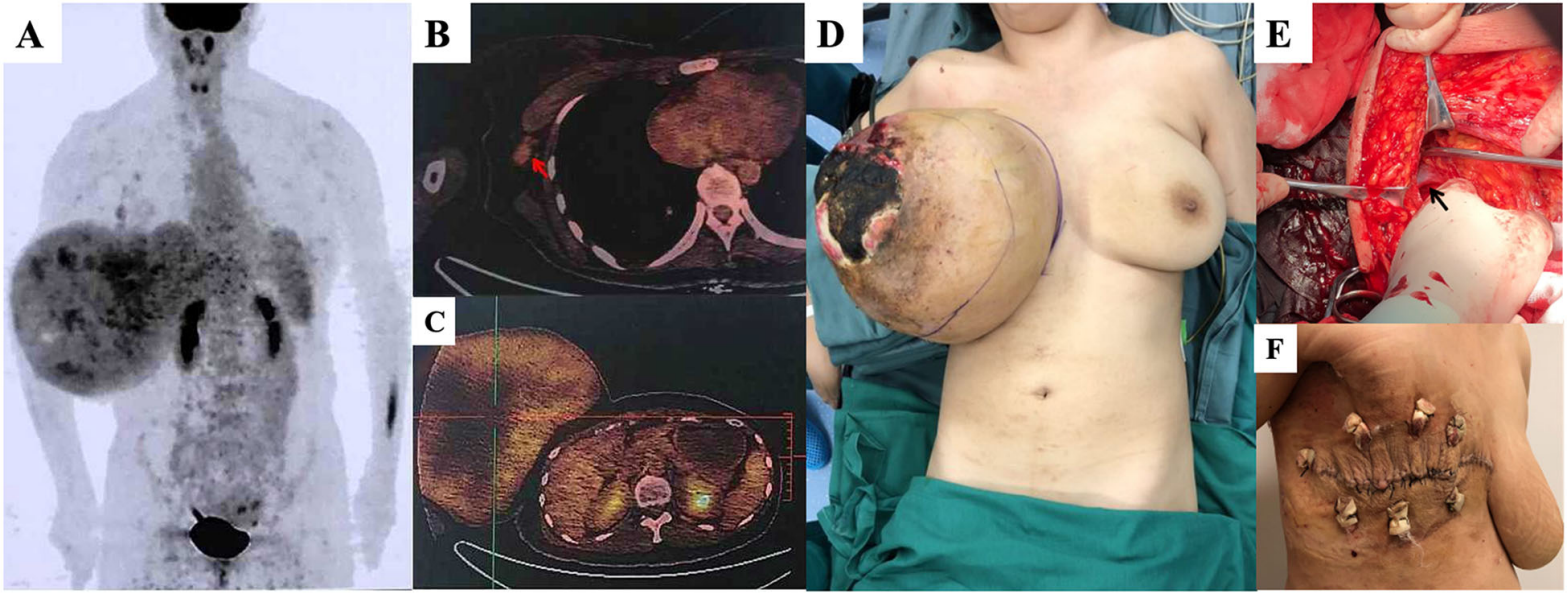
(A) A tumor with a maximum sectional area of 24 x 16cm by positron emission tomography/computed tomography (PET/CT) scan, with no abnormality in the lungs or bone. (B) Enlarged lymph nodes (red arrow) in the right axilla, measuring 0.4-1.2 cm and with a maximum standardized uptake value (SUVmax) of 2.1. (C) Uneven increase the SUV of the mass, with an SUVmax value of 3.4. (D) The patient was placed under general anesthesia in the supine position. A giant right breast tumor with skin ulceration is visible. (E) The axillary lymph nodes were dissected (black arrow: axillary vein). (F) Surface wound 14 days after surgery.
